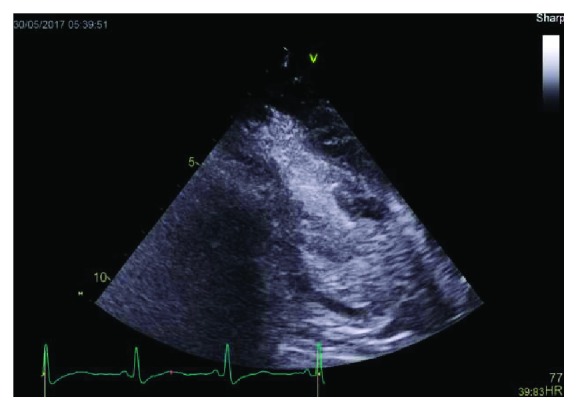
Apical 2 chamber view with agitated saline captured at end systole showing basal and apical ballooning with preserved contractility of the mid ventricular segments.
